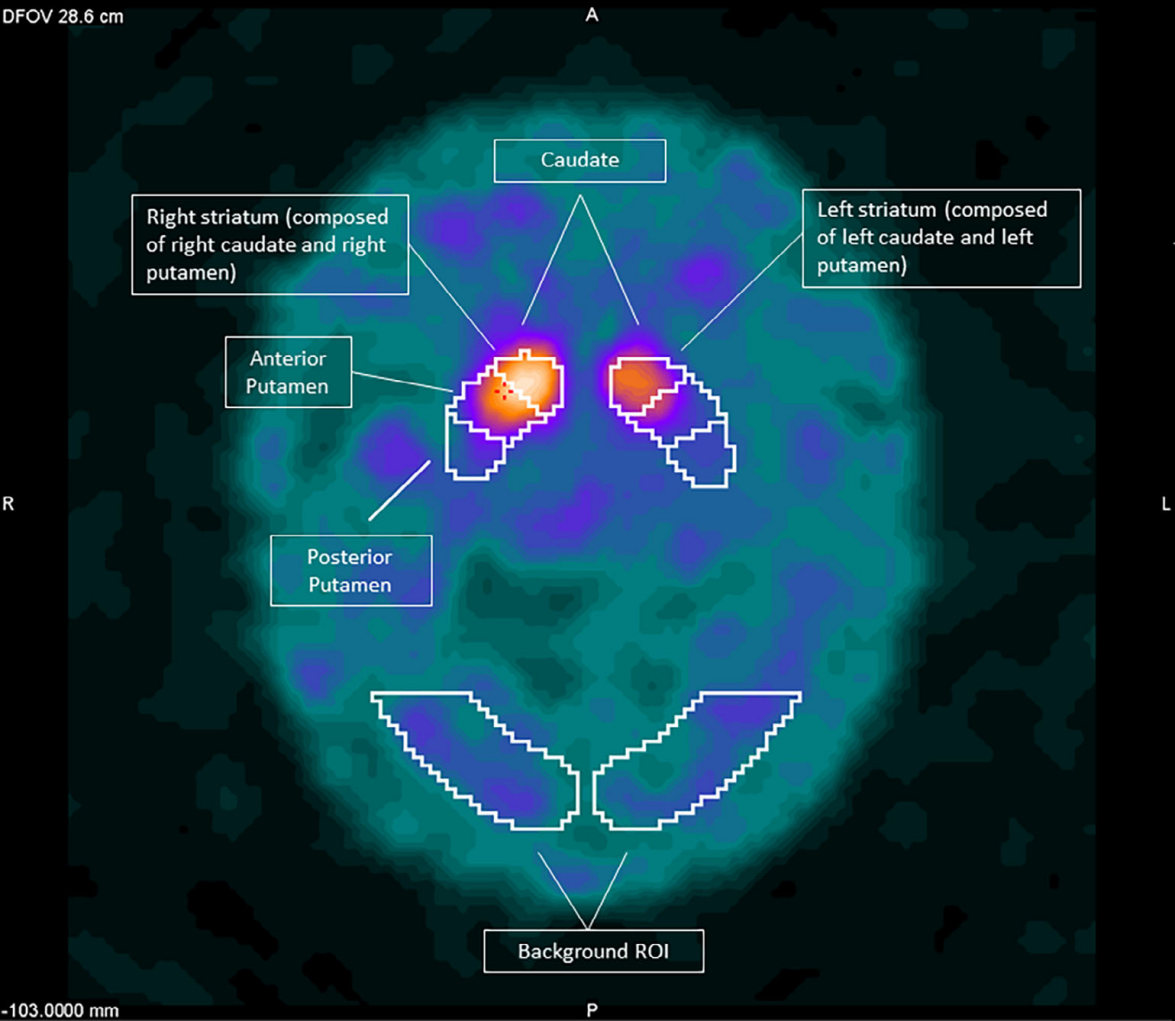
DaTQUANT SA volumes of interest (VOI) superimposed on a patient's automatically registered dopamine transporter single‐photon emission computed tomography (DAT‐SPECT) registered image.
